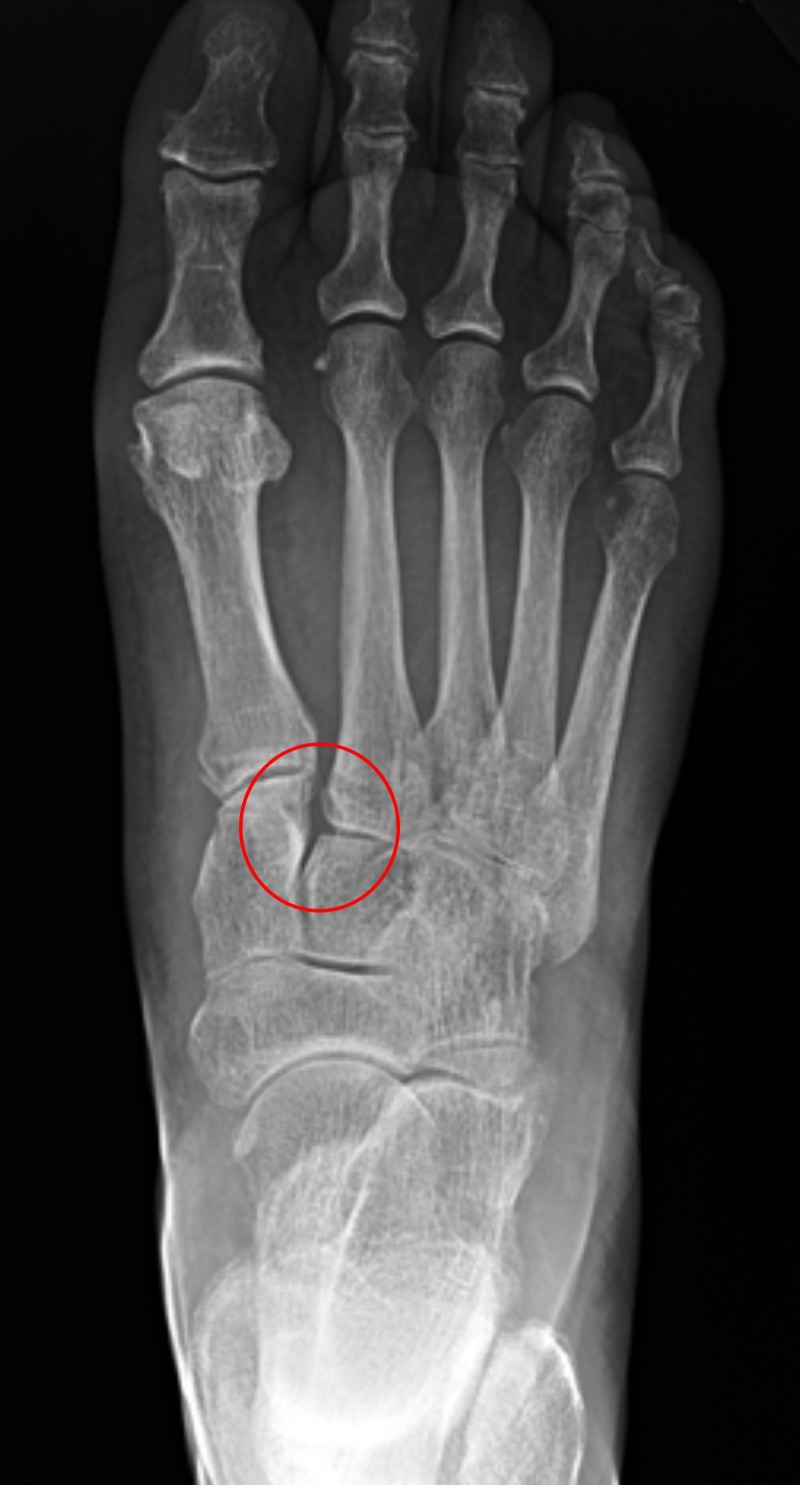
Red circle of the right foot X-ray highlights widening between the first and second ray, which is consistent with Lisfranc injury
